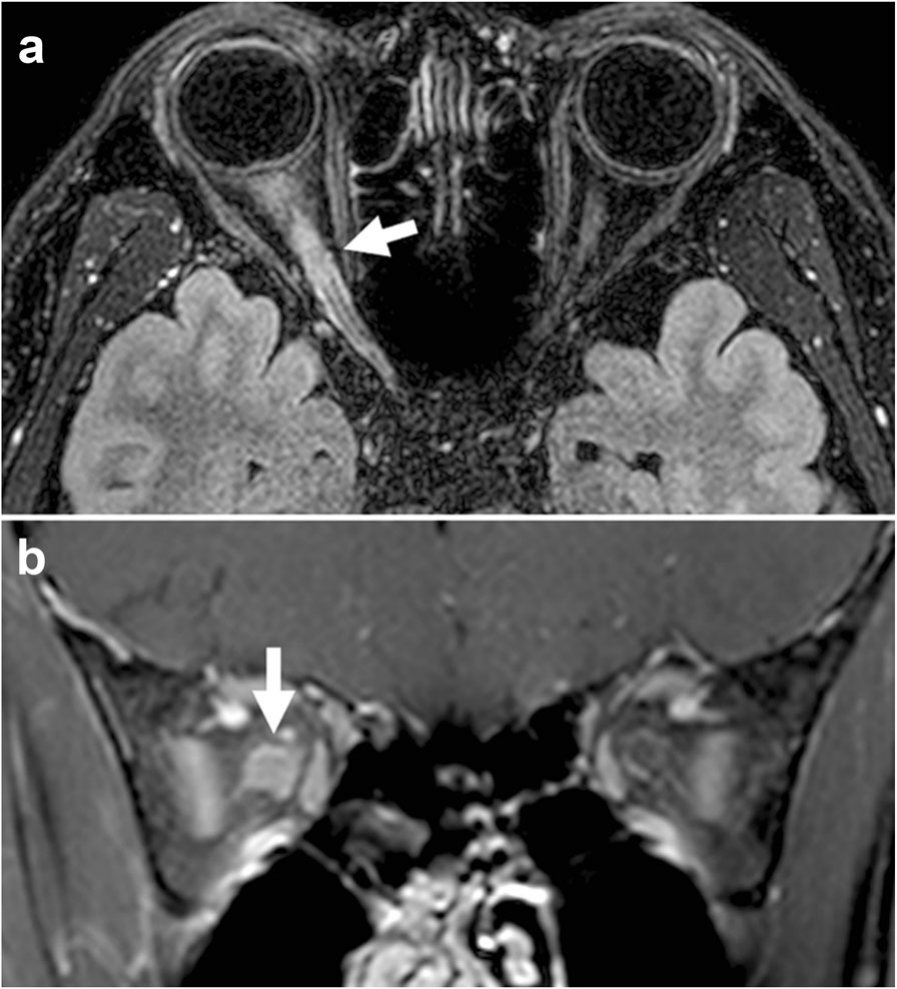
Contrast-enhanced three-dimensional fluid-attenuated inversion recovery-volume isotopic turbo spin echo acquisition (FLAIR-VISTA) axial image with fat suppression (a) and a contrast-enhanced T1-weighted coronal image with fat suppression (b) showing enhancement of the right optic nerve (arrows)
